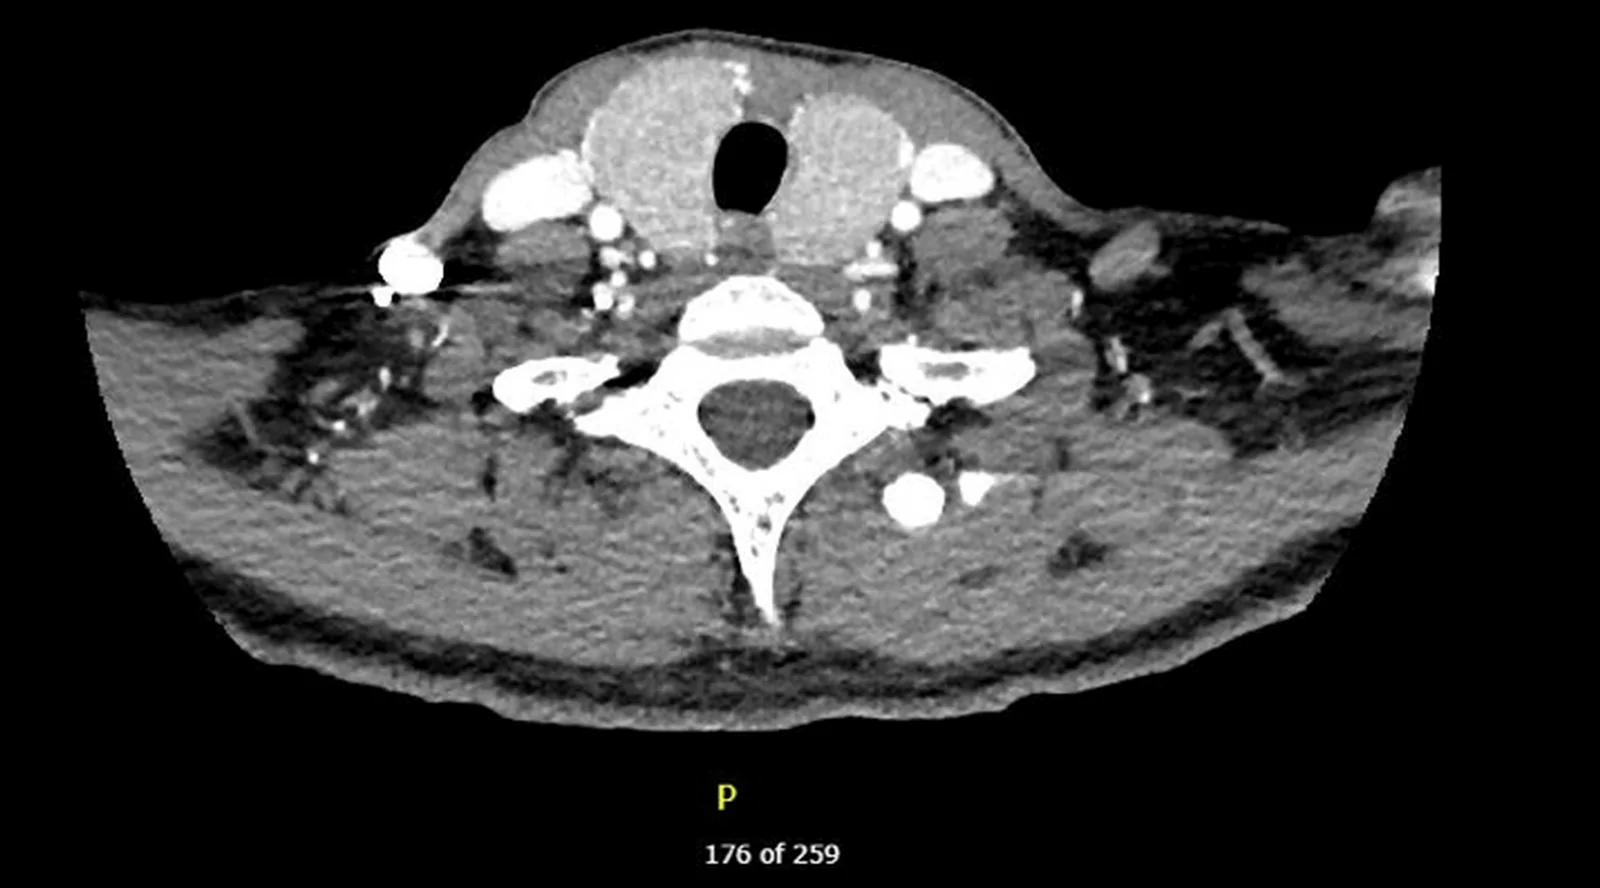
Axial contrast-enhanced CT, Year 4 (1/2021) Diffusely enlarged thyroid gland with multiple nodules, including a subcentimeter enhancing nodule in the right upper pole, and an isthmus thickness of 1.7 cm, extending from the hyoid bone to the superior border of the clavicles (right lobe 6.7 × 4.0 × 2.5 cm; left lobe 7.0 × 4.0 × 2.4 cm).
